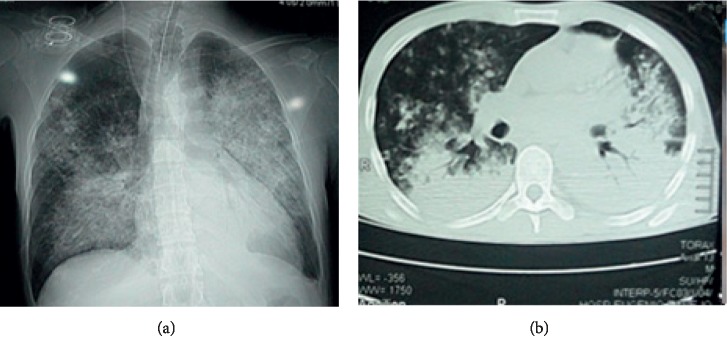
(a) Chest X-ray; AP view: diffuse infiltrate redistributed to the bases; (b) chest CT: diffuse bilateral alveolar infiltrate.
